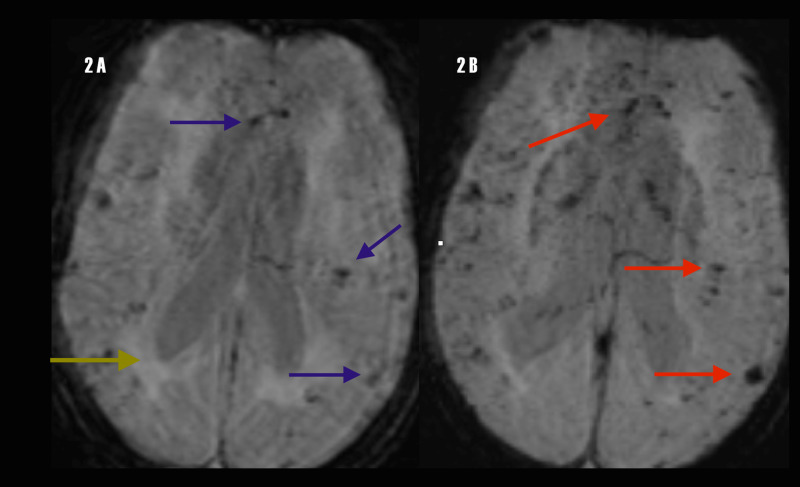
MRI: (A) Susceptibility weighted images (SWI) demonstrating hypointense CMBs (blue arrows). (B) Phase map revealing hypointense CMBs and betraying the right-handed reference frame of the MRI system (red arrows). These images look similar but are different acquisition sequences; note difference in peri-ventricular white matter intensity between both sequences (yellow arrow). MRI: Magnetic resonance imaging; CMB: Cerebral micro-bleeds; SWI: Susceptibility weighted images.
